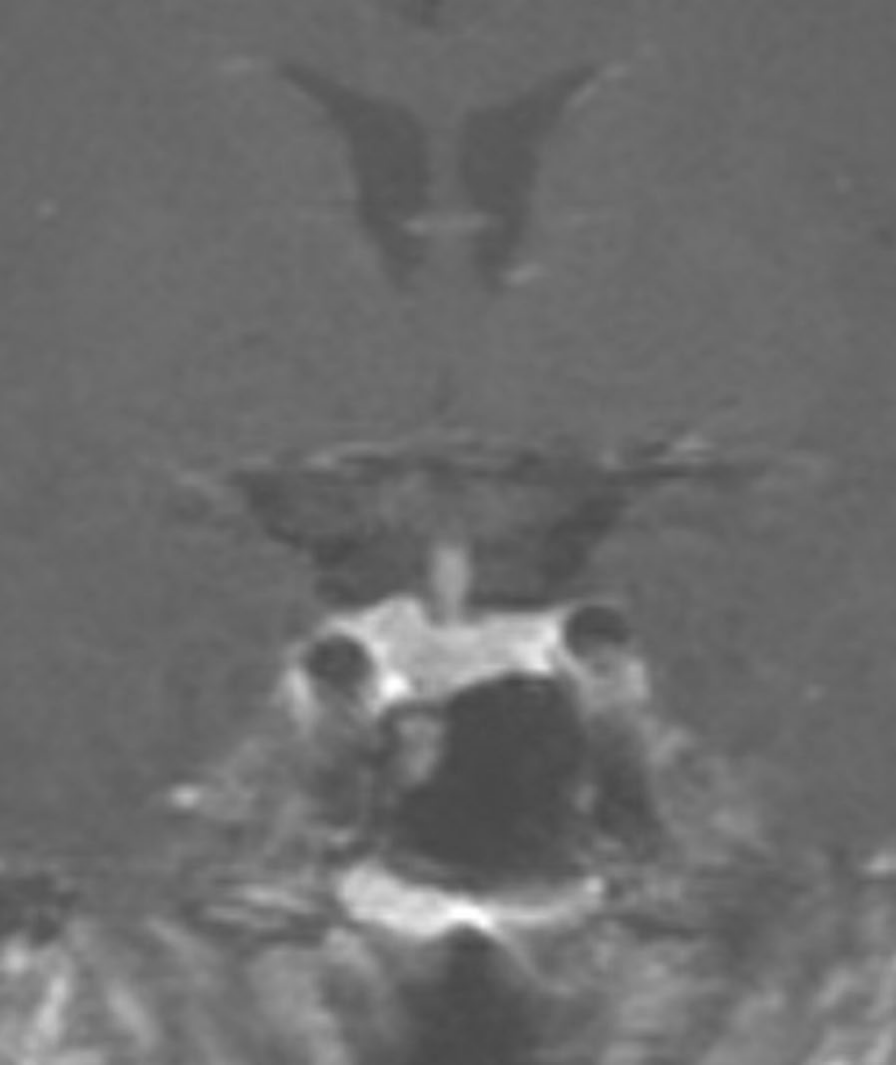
T1-weighted, dynamic 3T magnetic resonance imaging of the pituitary revealing subtle asymmetry involving the right aspect of the sella without focal lesion noted
